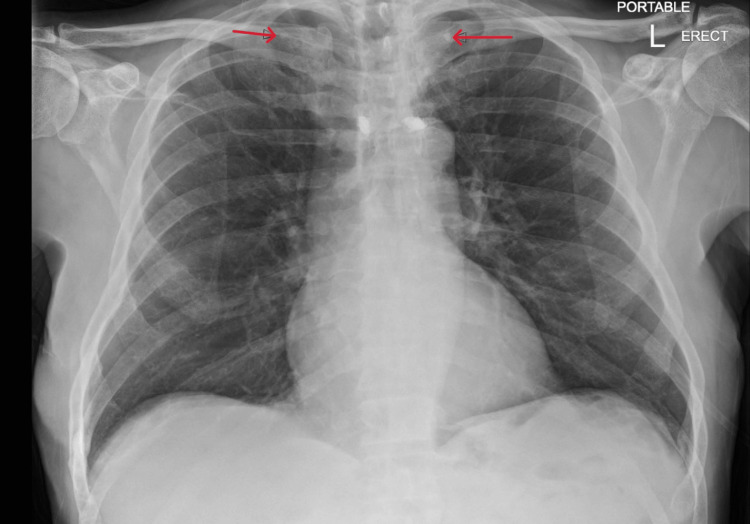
Chest X-ray showing new bilateral apical calcified granulomas
